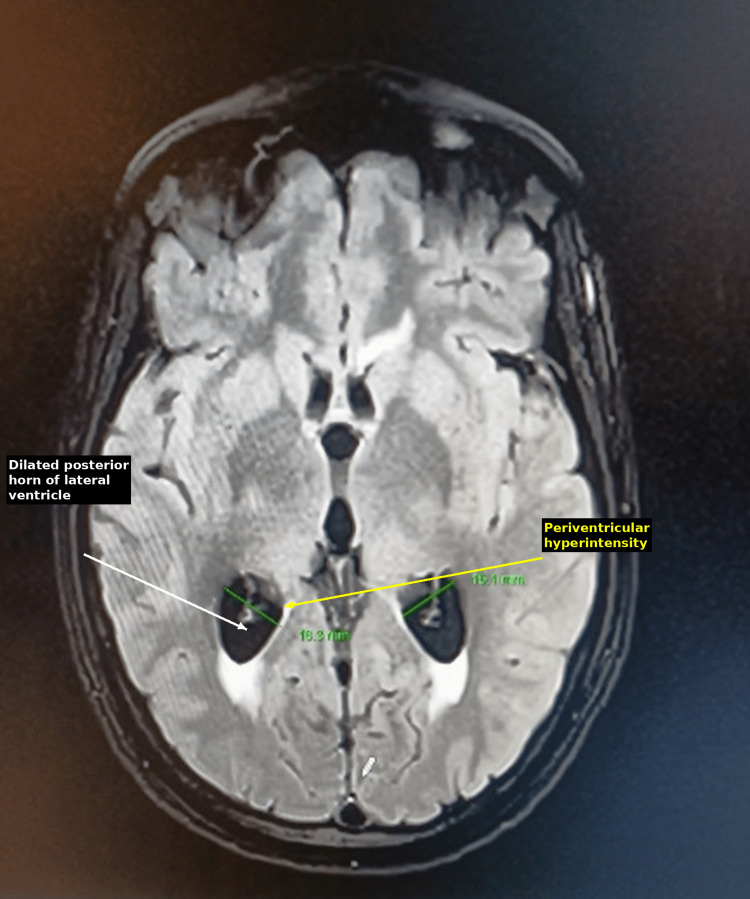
Axial FLAIR-weighted MRI of the brain demonstrating dilation of the posterior horn of the lateral ventricle (white arrow) with associated periventricular hyperintensity (yellow arrow), suggestive of periventricular flaring. No microinfarcts are identified in the cerebral parenchyma, basal ganglia, or brainstem MRI: magnetic resonance imaging, FLAIR: fluid-attenuated inversion recovery
